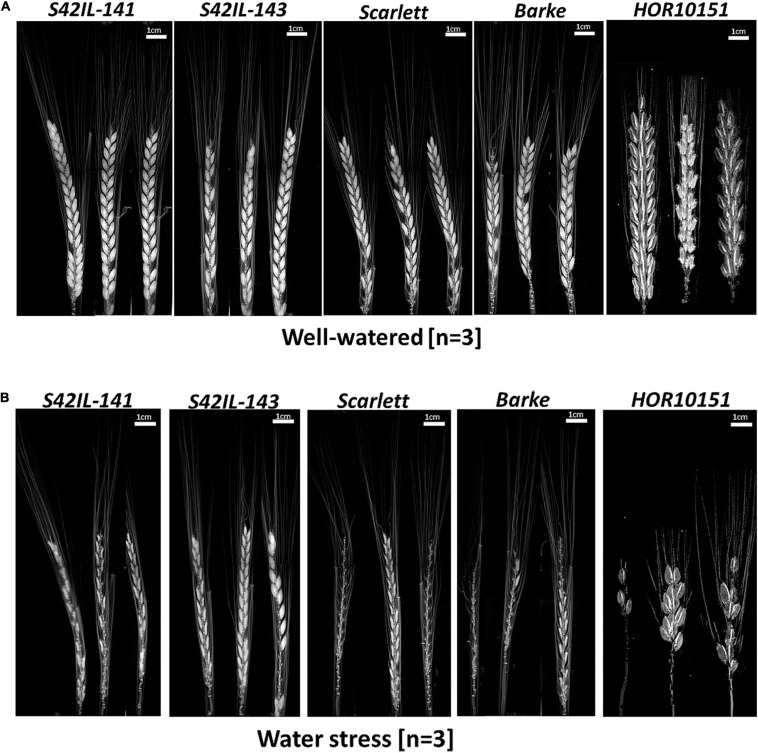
MRI amplitude images of barley main spikes at BBCH-scale 83, 15 days after stress application. (A) Main spikes of *S42IL-141, S42IL-143, Scarlett, Barke*, and *HOR10151* grown under well-watered conditions. (B) Spikes from plants grown under water stress (*n* = 3, scale = 1 cm).
